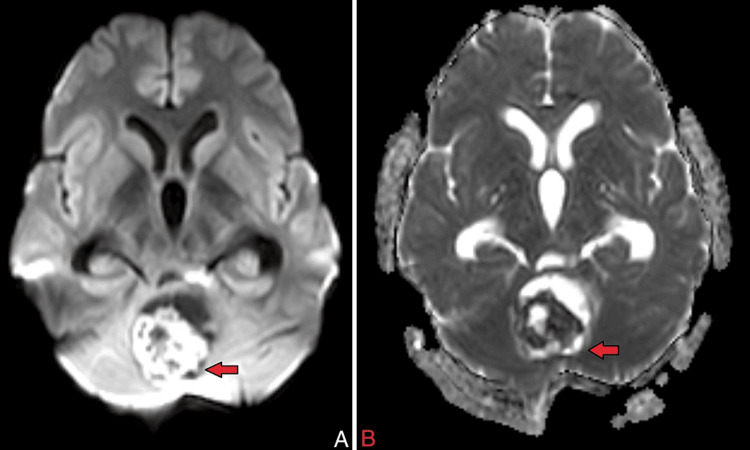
A) Axial DWI and B) ADC MRI brain images The red arrow shows that the lesion restricts DWI and corresponds to a low signal on ADC. DWI: Diffusion-weighted imaging; ADC: Apparent diffusion coefficient; MRI: Magnetic resonance imaging
